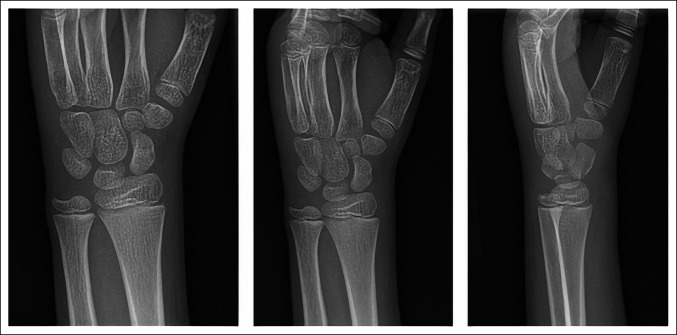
AP, oblique, and lateral radiographs of the left wrist 2 weeks before presentation to our emergency department.
